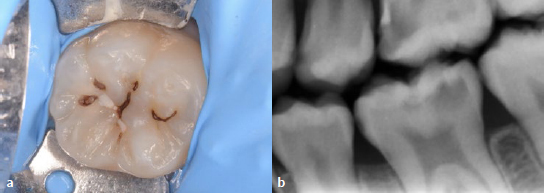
Preoperative examination of the tooth 36. a) Preoperative occlusal view. b) A deep carious lesion was detected in the bitewing.
